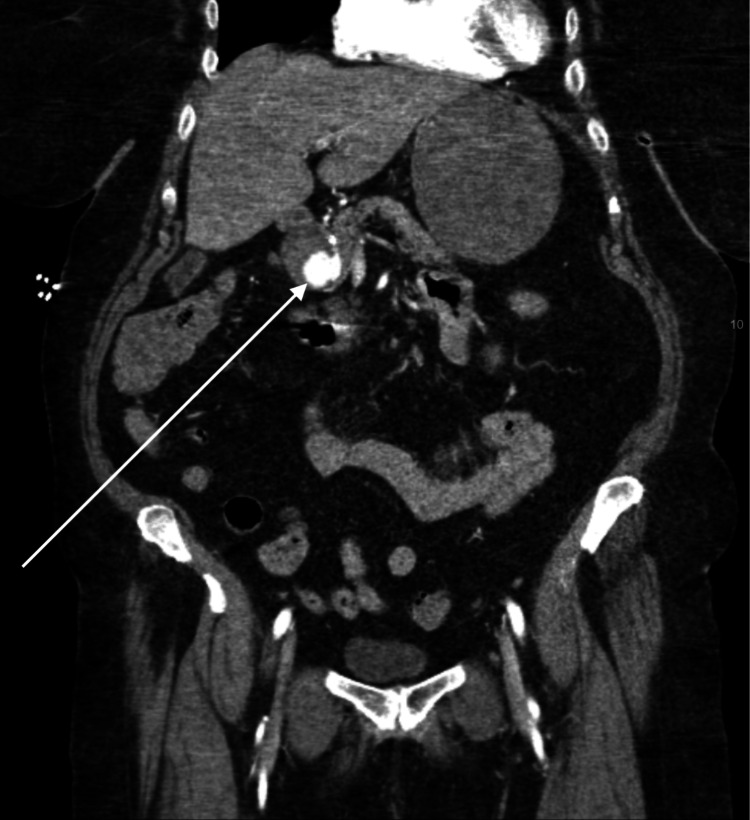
CTA (computed tomography angiogram) of abdomen and pelvis with extravasation from a branch of the celiac artery into a cystic space, found to be a large gastroduodenal artery pseudoaneurysm.
